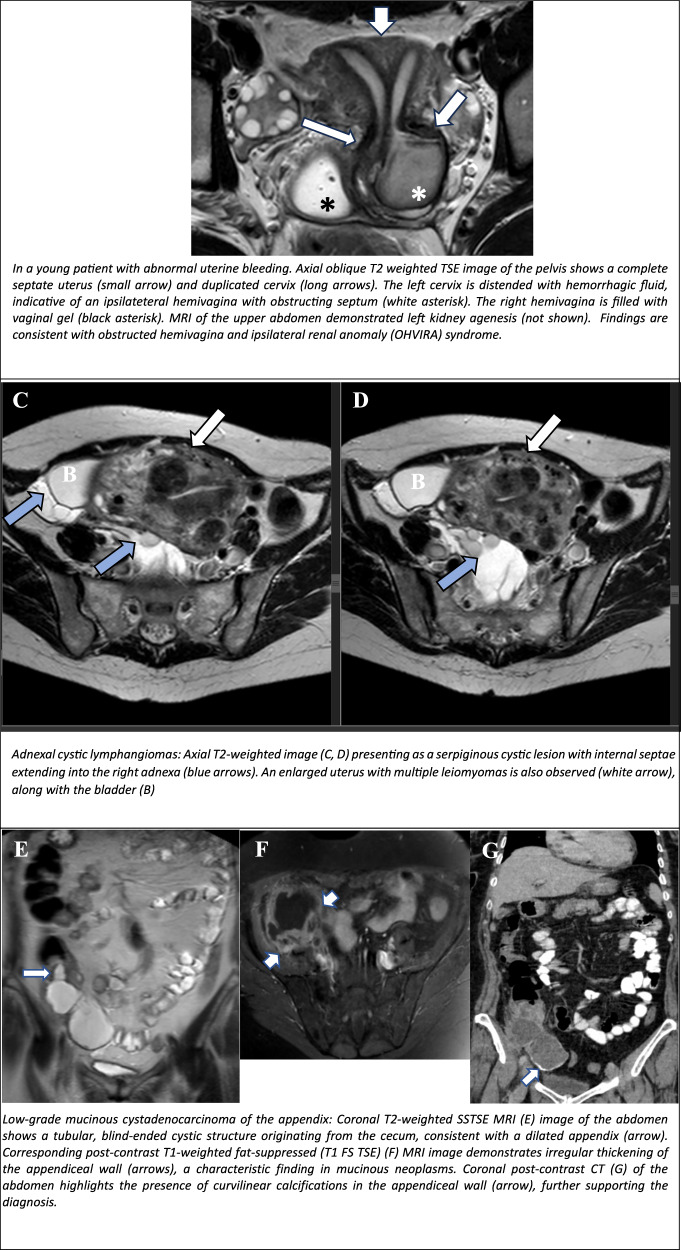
Non-ovarian cystic female pelvic lesions. PIC in a patient with chronic pelvic pain and a history of total colectomy due to ulcerative colitis. Coronal T2-weighted SSTSE image of the abdomen demonstrates a multilocular cystic lesion on the right pelvis (long arrow). Note the entrapment of the right ovary at the periphery of the lesion (small arrow), a highly indicative finding of PIC. A degenerative (hydropic) leiomyoma presented with abdominal distention (confirmed on surgical-pathological examination). Coronal T2-weighted TSE image (A) demonstrates a large mixed cystic–solids mass occupying the pelvis (white arrows). Note the presence of low T2 signal strands within the mass separated by fluid (fibre split sign, black arrows). Corresponding T2-weighted TSE image (B) in the sagittal plane shows a vascular pedicle (arrow) extending from the anterior uterine wall to the mass. The black arrow in (B) points to the uterus. Young girl with primary amenorrhoea and cyclic (monthly) pain. Coronal T2-weighted TSE image demonstrates haemorrhagic fluid within a dilated uterine cavity (hematometra, blue arrow) and vagina (hematocolpos, long white arrows). A low T2 signal line located at the level of introitus corresponds to the imperforated hymen (small white arrow). In a young patient with abnormal uterine bleeding. Axial oblique Τ2 weighted TSE image of the pelvis shows a complete septate uterus (small arrow) and duplicated cervix (long arrows). The left cervix is distended with haemorrhagic fluid, indicative of an ipsilateral hemivagina with an obstructing septum (white asterisk). The right hemivagina is filled with vaginal gel (black asterisk). MRI of the upper abdomen demonstrated left kidney agenesis (not shown). Findings are consistent with obstructed hemivagina and ipsilateral renal anomaly (OHVIRA) syndrome. Αdnexal cystic lymphangiomas: axial T2WI (C, D) presenting as a serpiginous cystic lesion with internal septae extending into the right adnexa (blue arrows). An enlarged uterus with multiple leiomyomas is also observed (white arrow), along with the bladder (B). Low-grade mucinous cystadenocarcinoma of the appendix: Coronal T2-weighted SSTSE MRI (E) image of the abdomen shows a tubular, blind-ended cystic structure originating from the caecum, consistent with a dilated appendix (arrow). Corresponding post-contrast T1-weighted fat-suppressed (T1 FS TSE) (F) MRI image demonstrates irregular thickening of the appendiceal wall (arrows), a characteristic finding in mucinous neoplasms. Coronal post-contrast CT (G) of the abdomen highlights the presence of curvilinear calcifications in the appendiceal wall (arrow), further supporting the diagnosis
